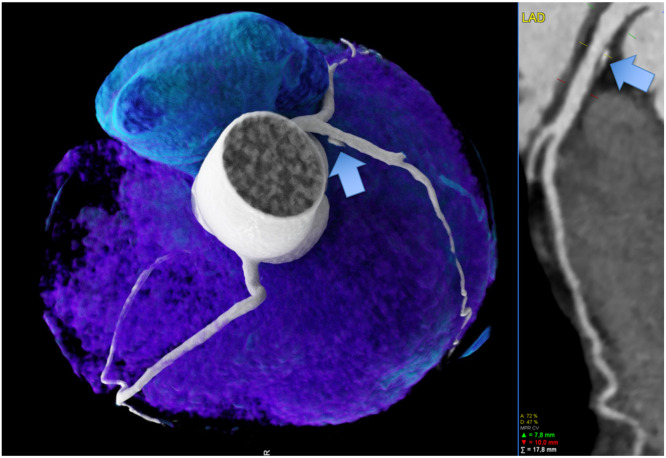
59 years-old-male cyclist with a high-level of training over more than 10 years, no chest pain but referral to coronary CTA due to polytopic ectopic ventricular extrasystole during exercise. CTA showed a predominantly calcified plaque in the proximal LAD (arrows) with less than 50 % stenosis (CADRADS 2). 1 CVRF: arterial hypertension. Left: 3D volume rendering technique (VRT) and right: curved multiplanar reformation (cMRP) showing the left anterior descending (LAD) coronary artery.
